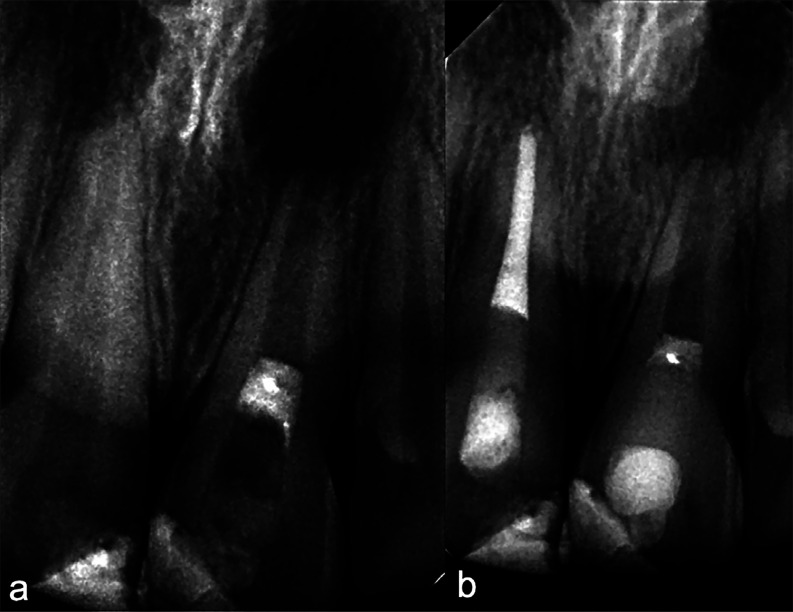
Postoperative and long-term periapical radiographic follow-up of tooth 21 after regenerative endodontic therapy. (a) Postoperative radiograph showing placement of the cervical MTA barrier over the collagen matrix/blood clot scaffold. (b) Four-year follow-up periapical radiograph showing resolution of the apical radiolucency and evidence of continued root development, including increased root length and hard tissue deposition in the middle and apical thirds.
